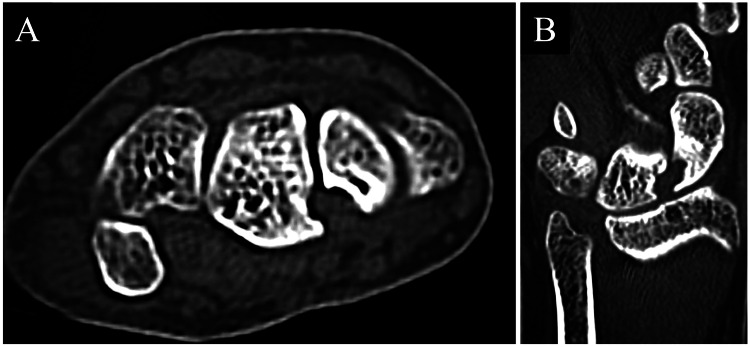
Computed tomography (CT) two years after the surgery. (A) Horizontal image of CT. (B) Coronal image of CT.
